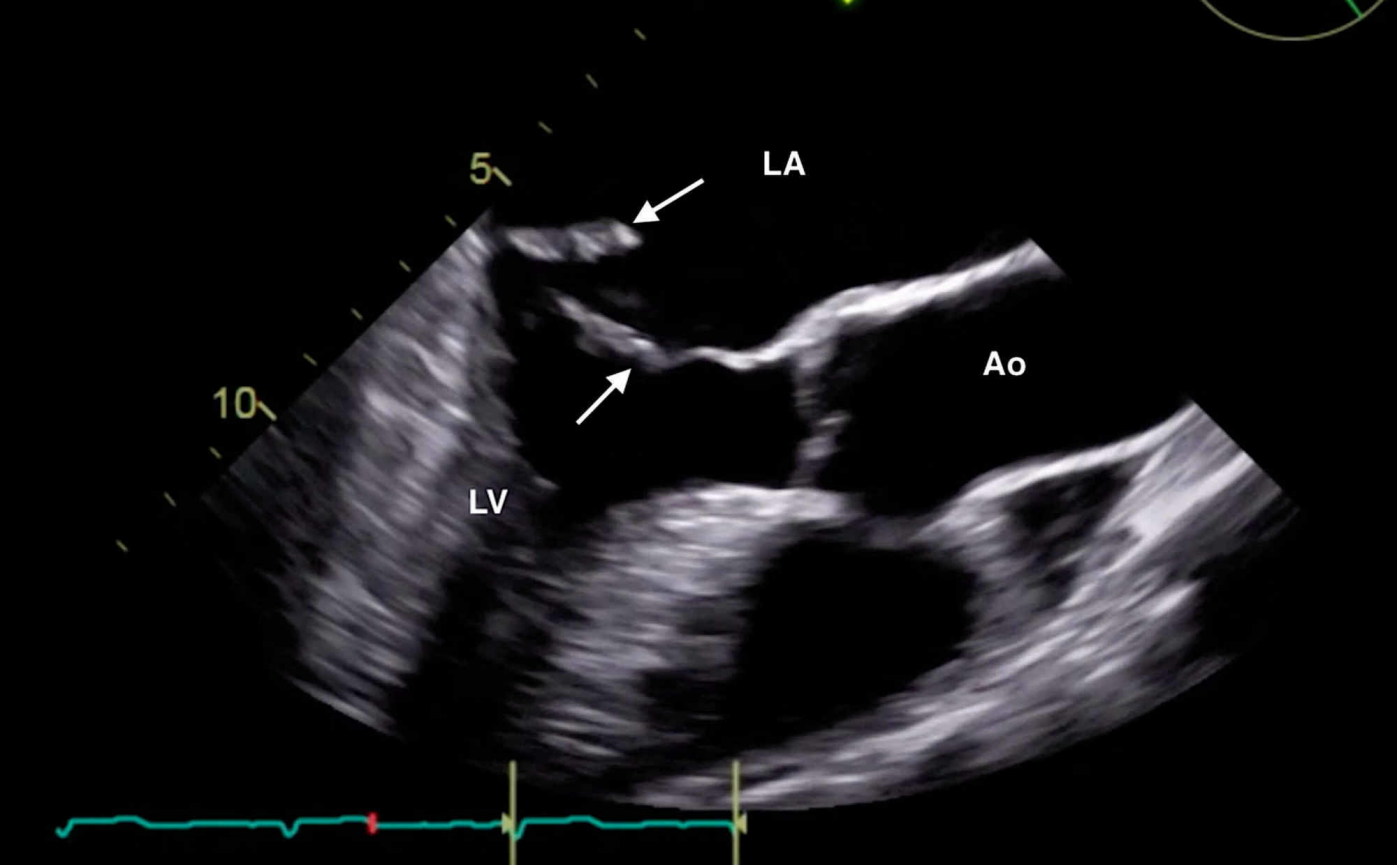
Transesophageal echocardiogram demonstrating normal position of the anterior mitral leaflet (rightward-pointing arrow) and flail motion of the posterior mitral leaflet (leftward-pointing arrow). Ao, aortic root; LV, left ventricle; LA, left atrium.
